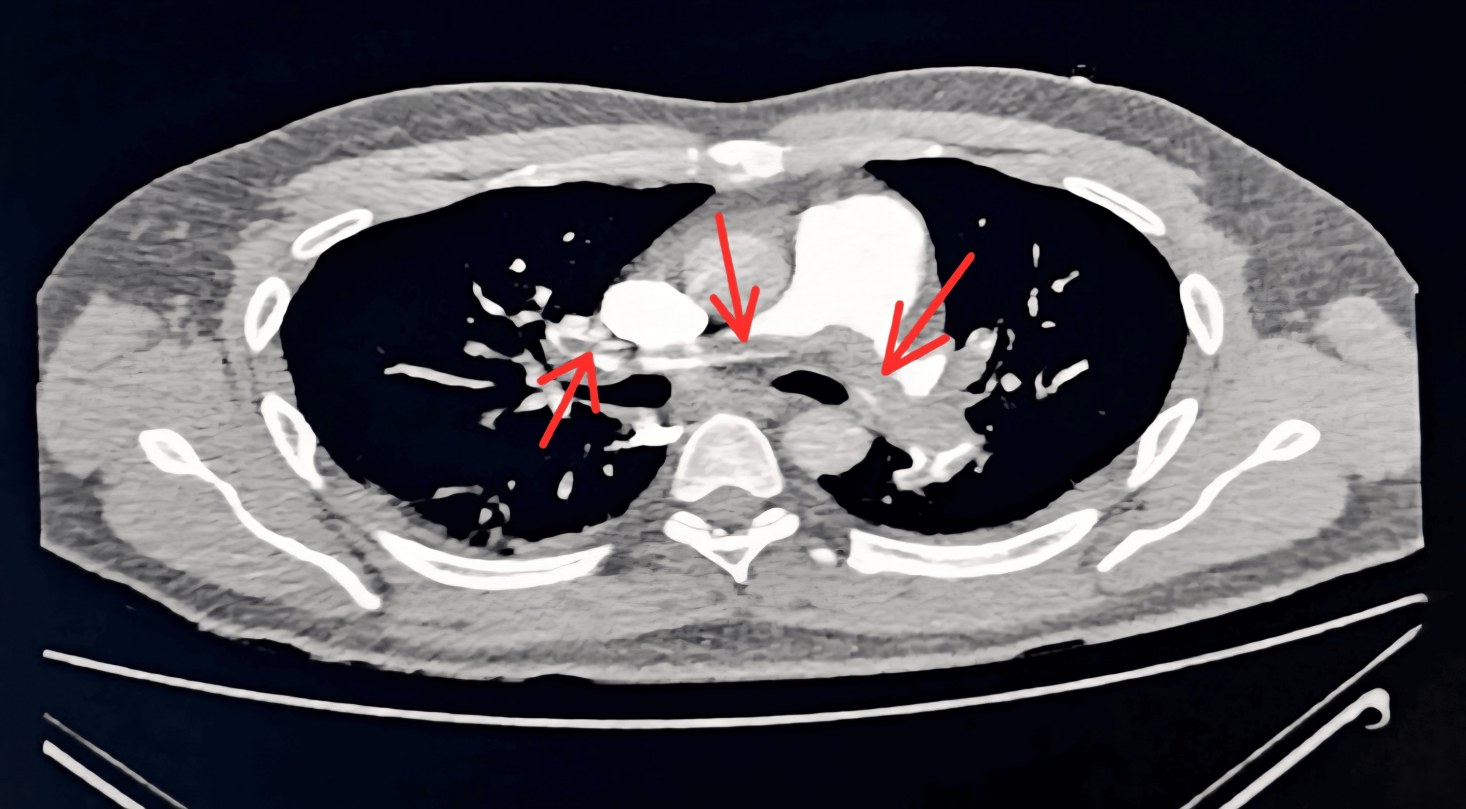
Chest CT scan showing a massive saddle pulmonary embolism. CT Pulmonary Angiogram showing a large saddle-shaped thrombus involving the right and left main pulmonary arteries with extension into segmental and subsegmental branches, consistent with massive pulmonary embolism
